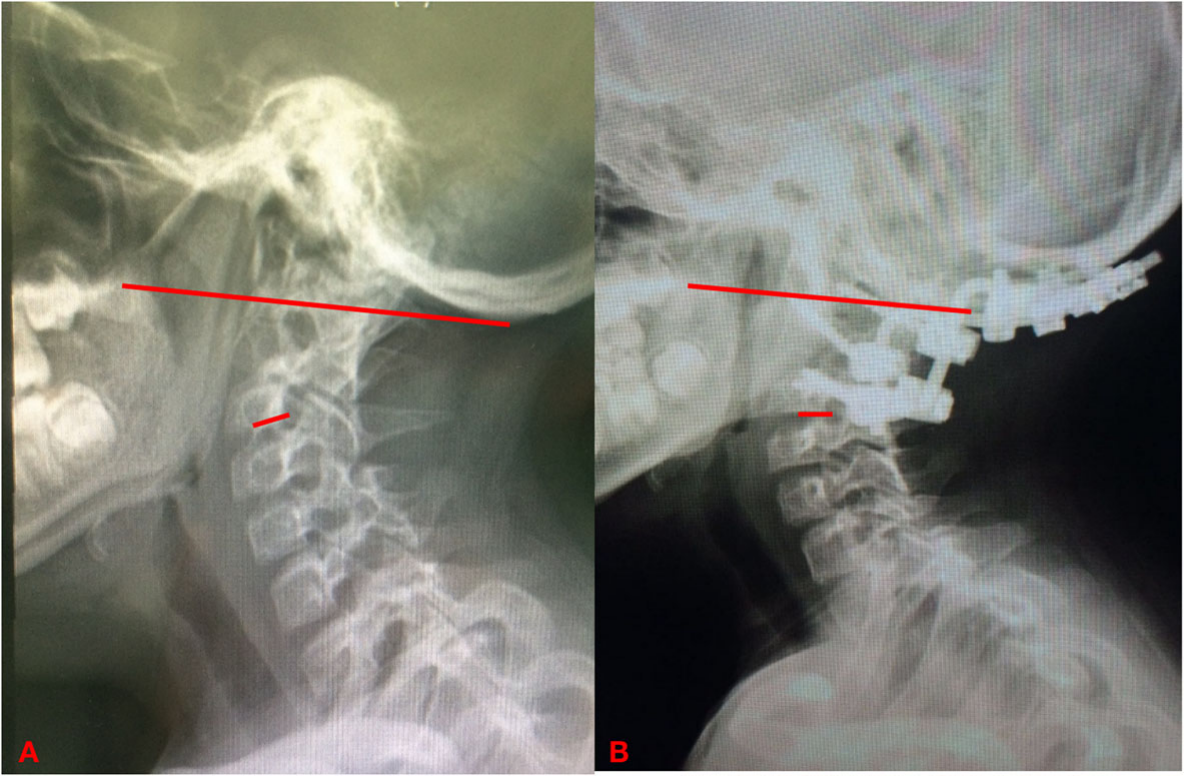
It is hard to make an accurate measurement of O-C4 angle as this patient had complex cervical abnormalities (congenital occipitoatlantal fusion, congenital basilar invagination, atlanto-axial joint dislocation, KFS at C2-3, and the subsequent fixation of O-C4). We made a rough estimation of the O-C4 angle (the angel between the long and short red lines) from lateral X radiography of preoperative (a) and postoperative (b) (2 months after surgery) in neutral position. A slight reflection of the O-C4 was noticed when compared with preoperative imaging (O, occiput; C, cervical vertebrae)
